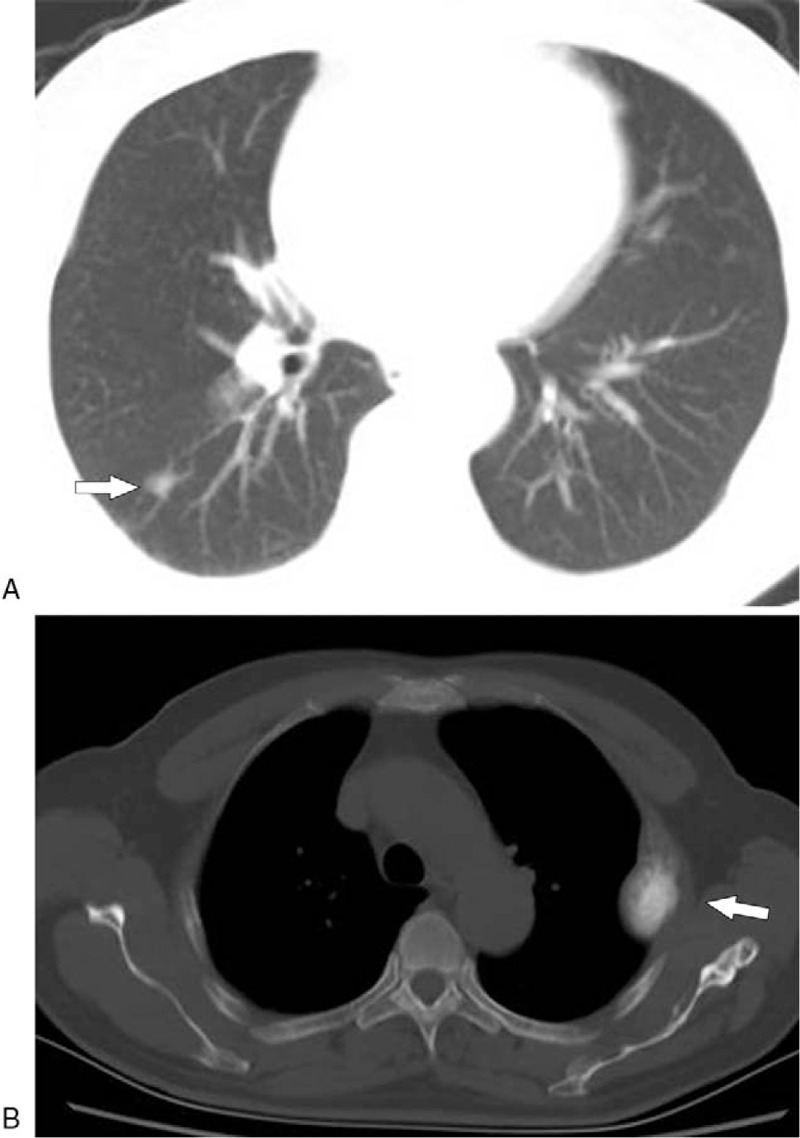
Axial: Chest CT scan shows a high-density nodule with spiculate boundary (arrow) (A). The bone window confirms the osteogenic changes of the third rib on the left side and the adjacent lung tissue being pushed (arrow) (B).
